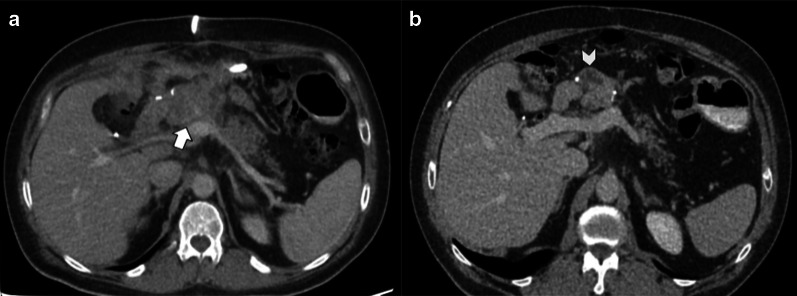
Collapsed loop versus Tumor recurrence. CT challenge. 58-year-old male patient with history of pancreaticoduodenectomy due to periampullary tumor. Control CT scans. (a) Heterogeneous, ill-defined image near pancreaticojejunostomy (big arrow) with peripheral fat stranding and contrast enhancement is seen after three months from surgery. No clinical symptoms or biological marker elevation were present. (b) 18 months CT scan control revealed total fat stranding resolution and demonstrated that the ill-defined enhancing image was due to a collapsed loop, which appears mild distended with normal fluid contain (arrowhead)
